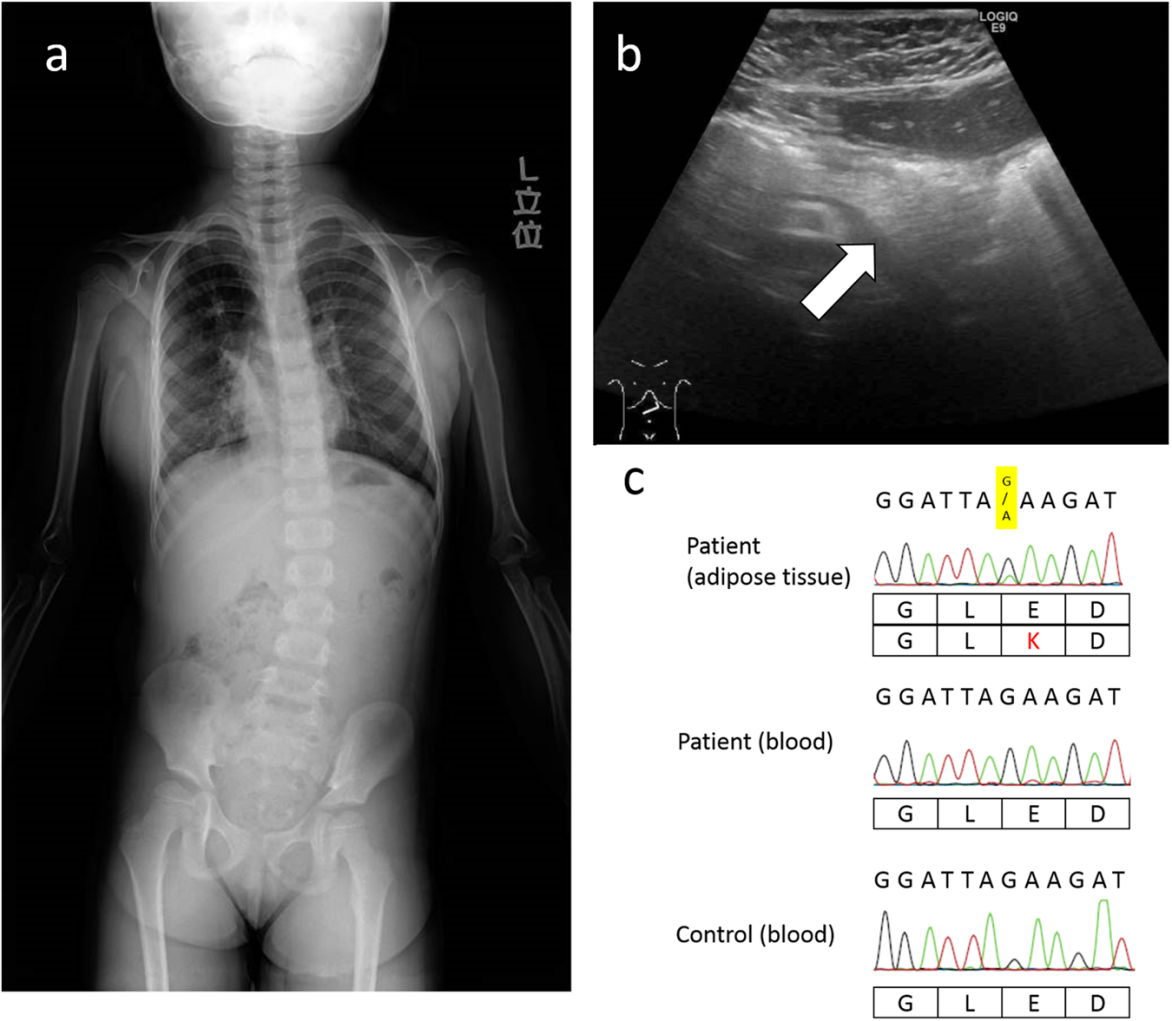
a Chest radiograph of the patient showed scoliosis. b Ultrasonography showed a hyperechogenic pancreas (white arrow). c Results of *PIK3CA* gene analysis by Sanger sequencing. Identification of a mosaic missense variant of *PIK3CA* (c.1357G>A causing p.Glu453Lys) in DNA derived from skin fibroblasts; no variant was observed in DNA derived from peripheral blood
